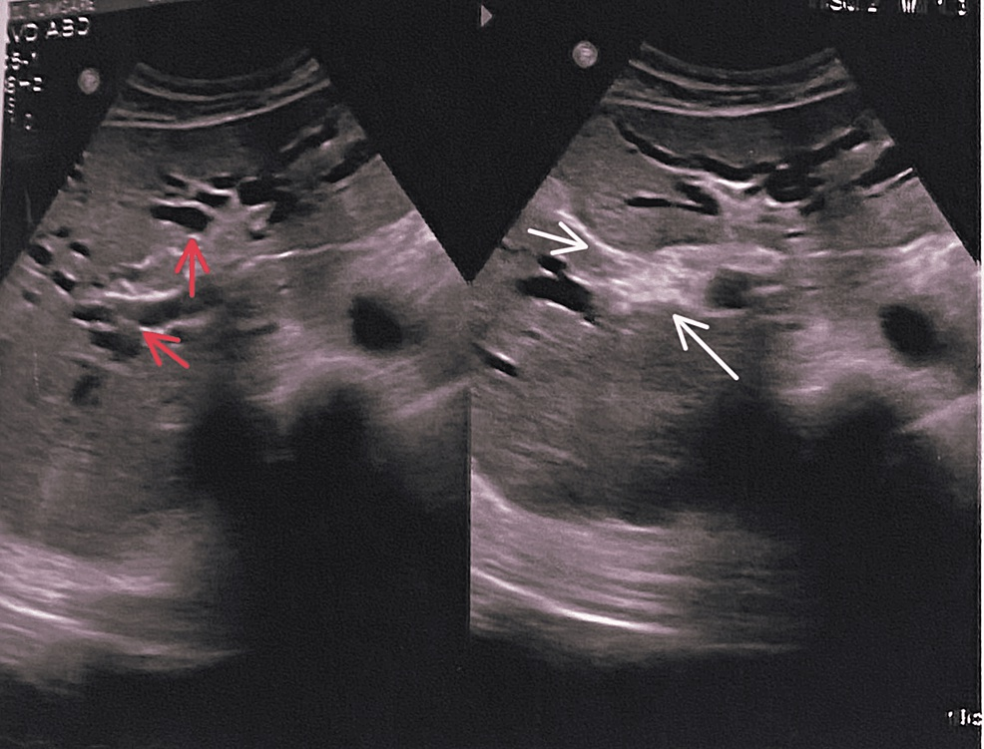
Ultrasonography of the whole abdomen Red arrows represent the dilated veins while the white arrow shows the obstructive dilatation of intrahepatic biliary radicals
